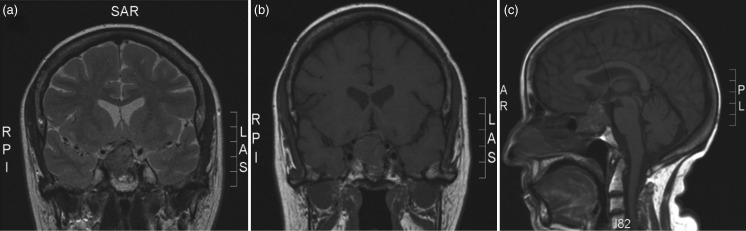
2008 MRI pituitary: (a) coronal T2WI, (b) coronal T1WI and (c) sagittal T1WI respectively.
